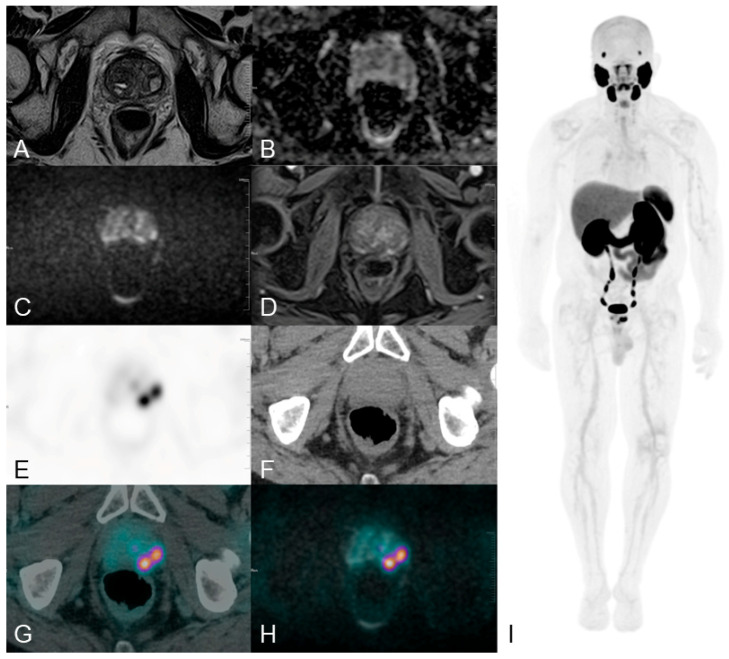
MRI and PSMA-PET/CT in prostate cancer staging. Long Axial Field-of-View (LAFOV) Total-Body PET/CT (194 cm uEXPLORER, United Imaging, Shanghai, China) with 18F-Piflufolastat (2.64 MBq/kg) in a patient with Gleason 8 (4 + 4) and PSA 14.2 ng/mL. Panels: (A) T2-weighted MRI; (B) ADC; (C) high b-value DWI; (D) contrast-enhanced T1; (E) attenuation-corrected PSMA-PET; (F) low-dose CT; (G) fused PET/CT; (H) PET/MRI-DWI fusion; (I) PET MIP. PET tracer distribution is visualized using the ‘Warm-Metal’ linear color scale. Image reconstruction and post-processing were performed using United Imaging Healthcare PET/CT and CT Image Post-processing Software (uWS-MI:R002.32.0.1749877; uWS-CT:F006.34.0.1749877). Higher metabolic activity or tracer avidity is represented by the transition from cooler tones (black/green) to warmer tones (orange/white). MRI shows a left-lobe lesion with mild diffusion restriction; PET identifies two tracer-avid areas, including a posterior focus near the capsule and rectal wall not clearly seen on MRI. MRI and PET complement each other for accurate local staging. Images courtesy of San Pietro Fatebenefratelli Hospital, Rome.
